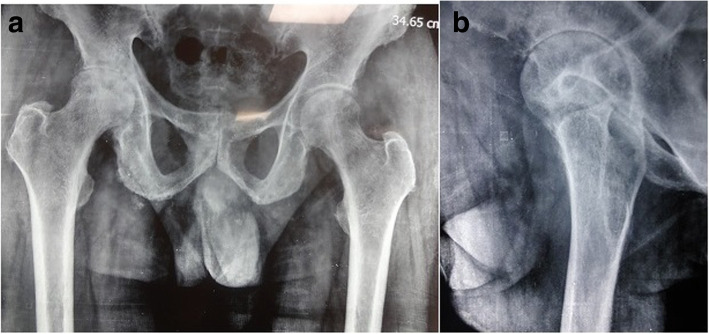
a & b Anteroposterior and lateral X-ray films of the pelvis, with both hip joints showing reduced join space with degenerative changes, irregularity, and flattening of femoral head
